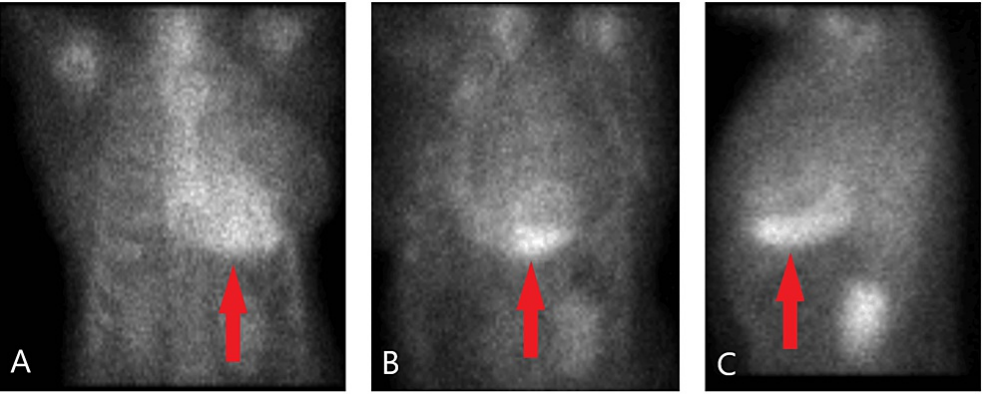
Planar technetium-labeled cardiac scintigraphy showing Grade 3 (severe uptake - heart greater than bone) (A) Anterior projection, (B) anterolateral projection, (C) lateral projection
